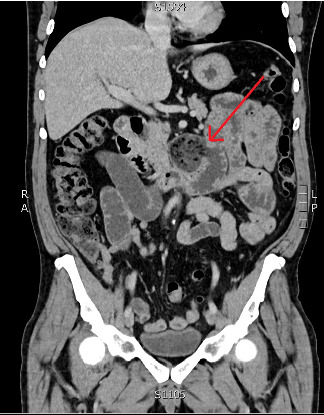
CT coronal view with arrow pointing to a large proximal jejunal diverticulum containing mixed densities without any evidence of inflammation.
